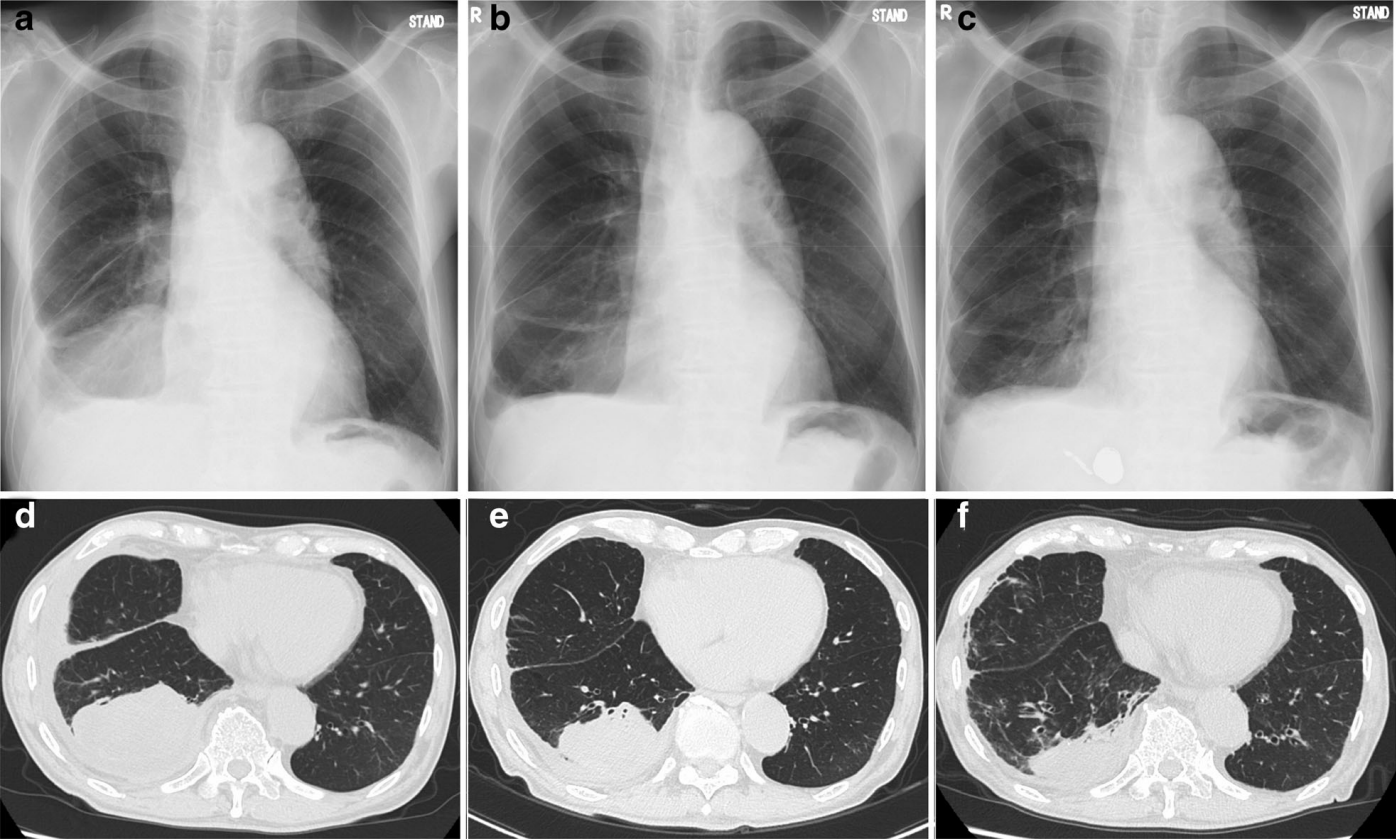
Chest X‐rays and computed tomography scans obtained (a) (d) before and (b) (e) two weeks and (c) (f) seven weeks after the start of the dabrafenib plus trametinib treatment.
